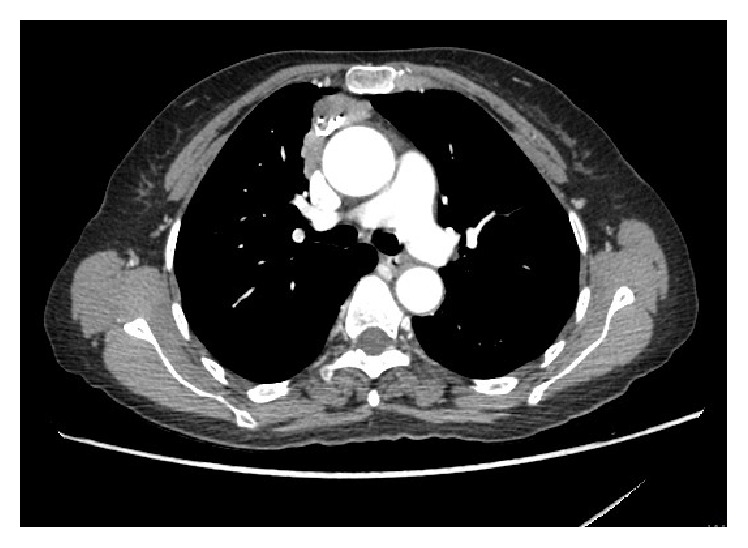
Chest CT showing anterior mediastinal mass with calcification and regional invasion consistent with athymic tumor.
